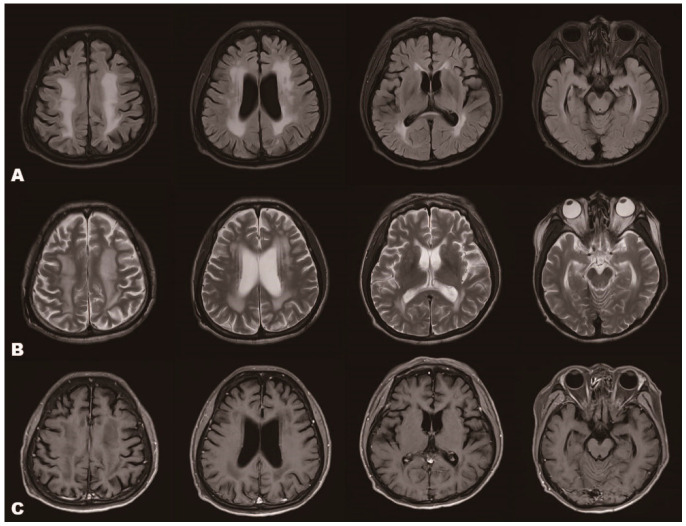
Brain magnetic resonance imaging of the patient. (A) Axial fluid-attenuated inversion recovery (FLAIR) images. (B) Axial T2-weighted images. (C) Axial T1-weighted images.
